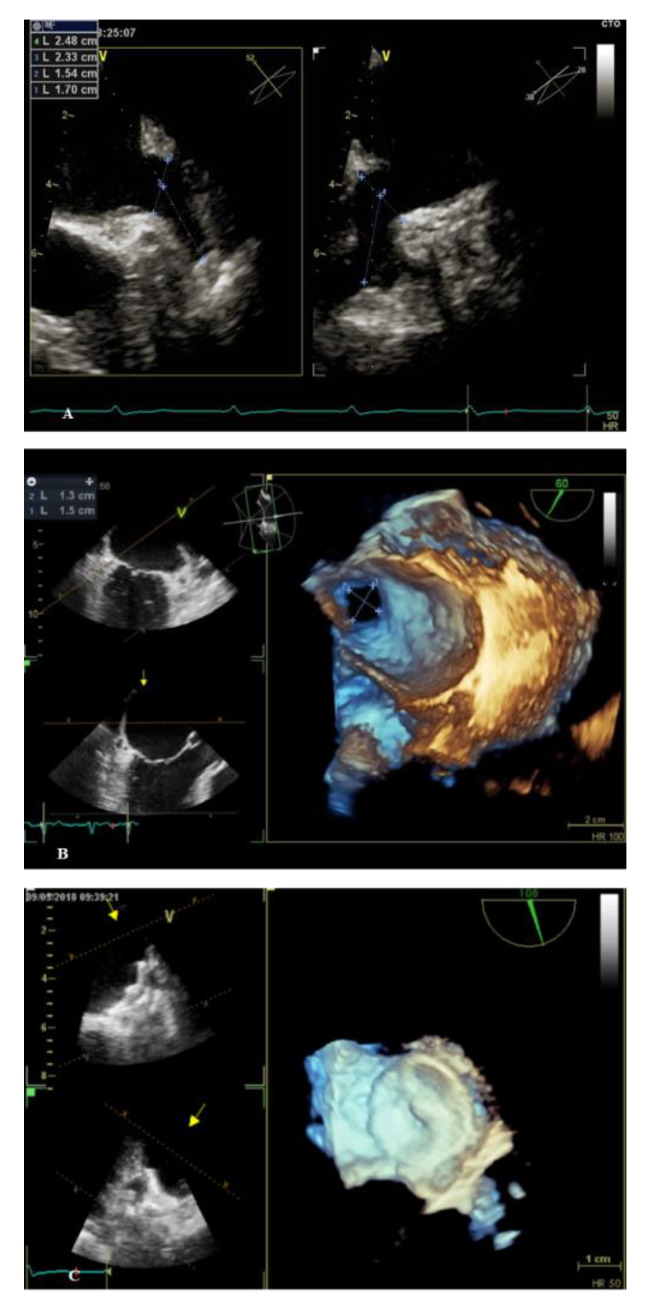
Examples of LAAO evaluation by 2D and 3D transesophageal echocardiography performed in our unit. (A) 2D-TEE evaluation of LAA; (B) 3D-TEE assessment of LAA; (C) 3D-TEE evaluation of an implanted LAA device.
